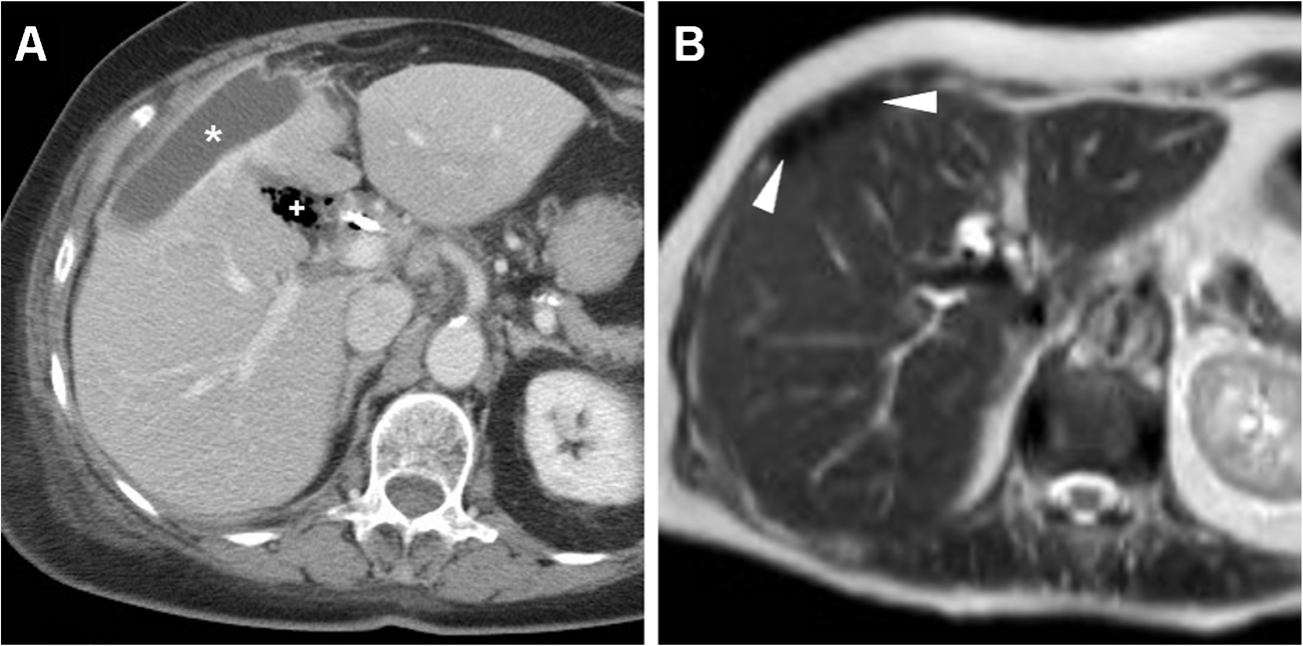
Perihepatic abscess diagnosed 10 days after endoscopic stent positioning through a cytologically benign distal CBD stricture in a 78-year-old female. Contrast-enhanced CT (a) requested because of fever and right hypochondrium pain revealed a fluid-like collection (*) with an enhancing peripheral rim and minimal extraluminal air (+) predominantly in the right-sided retroperitoneum. Urgent surgery included cholecystectomy and abscess drainage. Postoperatively, unenhanced T2-weighted MRI (b) depicted resolution of the abscess (*arrowheads*)
